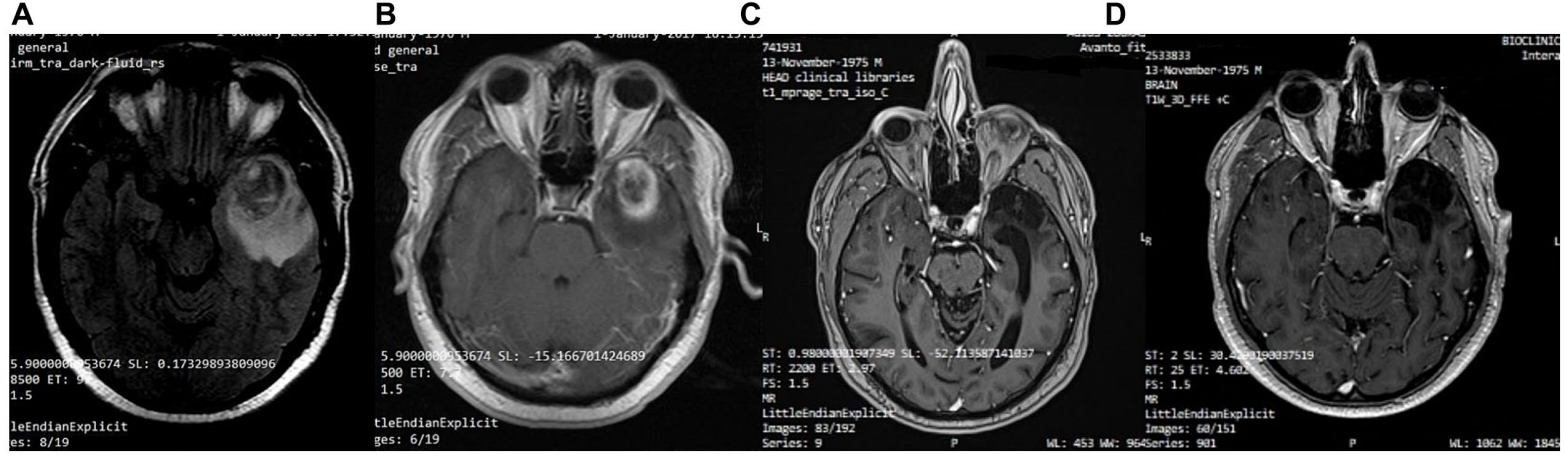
Patient 1: (A) Pre-operative brain MRI (T2/FLAIR) (B) Pre-operative brain MRI (T1 with contrast) (C) 38-month follow-up brain MRI (T1 with contrast) (D) 80-month follow-up brain MRI (T1 with contrast).
